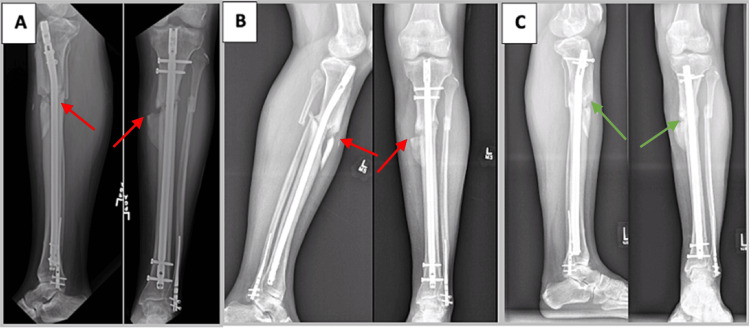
A 30-year-old man with oligotrophic tibial nonunion (case 1) A) Radiographs of the patient’s left leg taken eight months after injury demonstrating a nonunited (red arrows) and deformed tibia. B) Persistent nonunion (red arrows) 12 months following the patient’s second exchange nailing and allogeneic bone grafting. At this time, priority was placed on achieving union through a third exchange nailing rather than concomitantly addressing the tibia’s residual deformity. C) Tibial union (green arrows) is evident on radiographs taken one year after performing our modified exchange nailing operation utilizing cortical vent tunnels. The patient has since declined to proceed with tibial malunion correction surgery.
